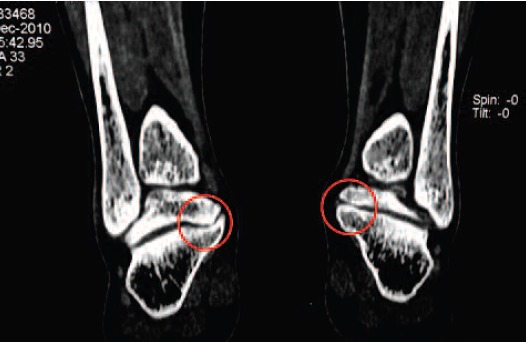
CT scan of the 2nd patient showing prominent medial
